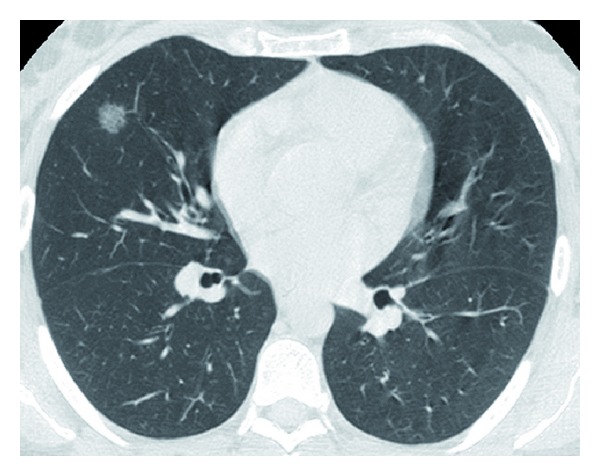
HRCT at the level of the middle lobe shows a 1.4 cm ill-defined nodule of a ground-glass opacity in the right middle lobe demonstrating air alveologram.
